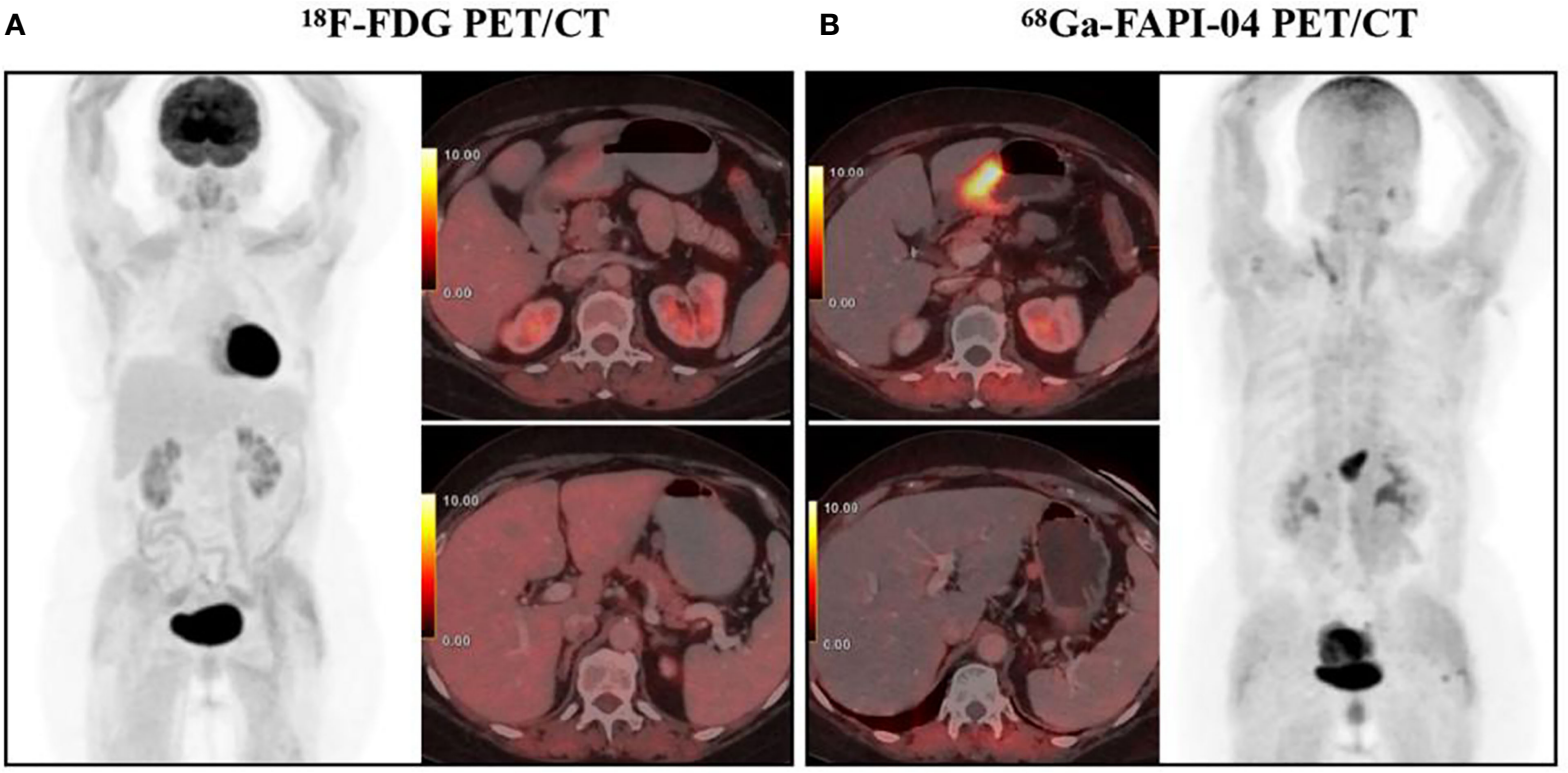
A 65-year-old woman diagnosed with poorly differentiated gastric adenocarcinoma underwent ^18^F-FDG PET/CT (A) and ^68^Ga-FAPI-04 PET/CT examination (B) respectively, showing a FAPI-positive, FDG-negative primary tumor (SUV_max_ 11.8 and 2.3, respectively) and a perigastric lymph node (SUV_max_ 2.3 and 0.3, respectively). With permission from Kuten et al. (49).
